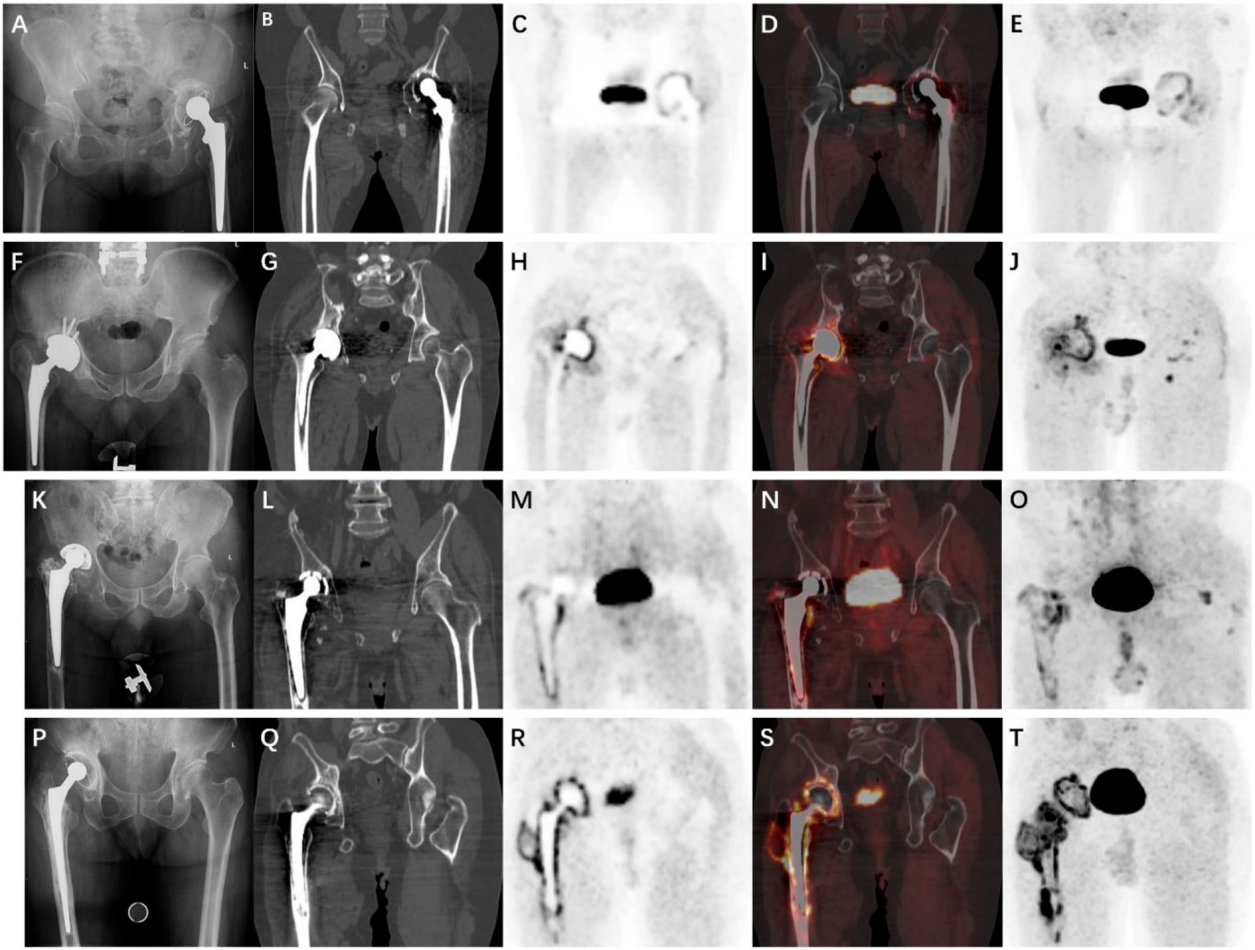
X-ray and ^68^Ga-FAPI PET/CT [gallium-68 (^68^Ga)-labeled fibroblast activation protein inhibitor positron emission tomography/computed tomography] images of each patient. (A–E) Case 1. (F–J) Case 2. (K–O) Case 3. (P–T) Case 4. First column: X-rays. Second column: Computed tomography (CT) images. Third column: Positron emission tomography (PET) images. Fourth column: Fusion images. Fifth column: Maximum intensity projection (MIP).
